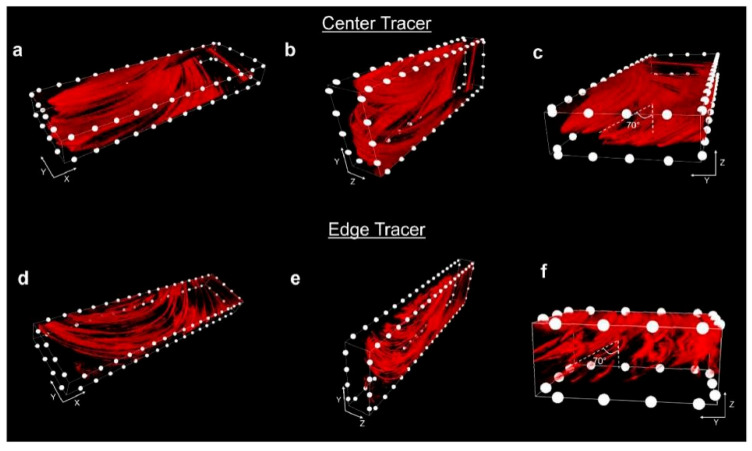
High-resolution CT results following steady-state deposition for (a–c) center tracers and (d–f) edge tracers. The spacing between each white dot is 1.25 mm [16].
